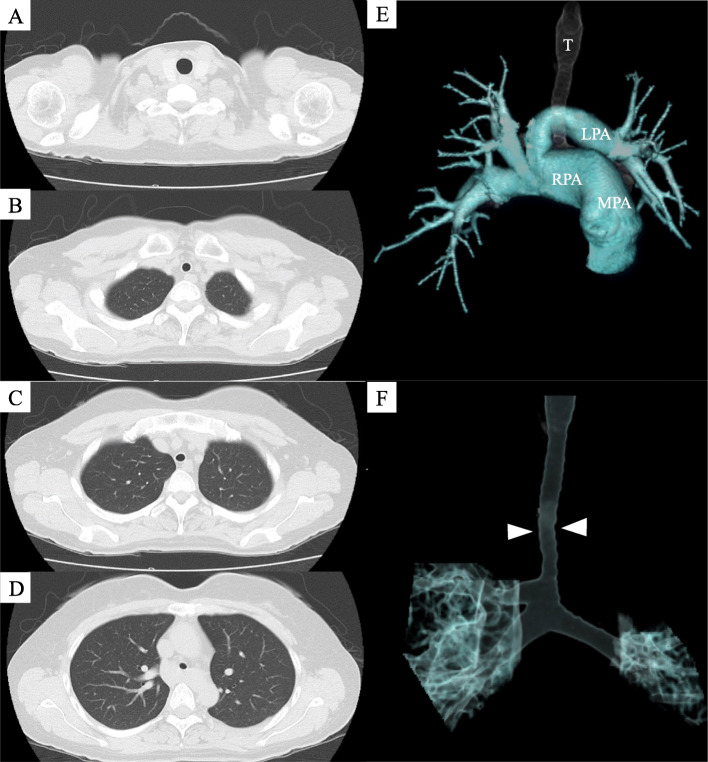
Preoperative imaging findings. Chest computed tomography showed stenosis over the entire length of the trachea with complete tracheal rings (A-D). Reconstructed three-dimensional images showed a pulmonary artery sling (E) and tracheal bronchus (F). The narrowest part of the trachea (arrowheads) was 6 mm in diameter (F). The left pulmonary artery originated from the right pulmonary artery and encircled the trachea (E). MPA, main pulmonary artery; RPA, right pulmonary artery; LPA, left pulmonary artery; T, trachea
